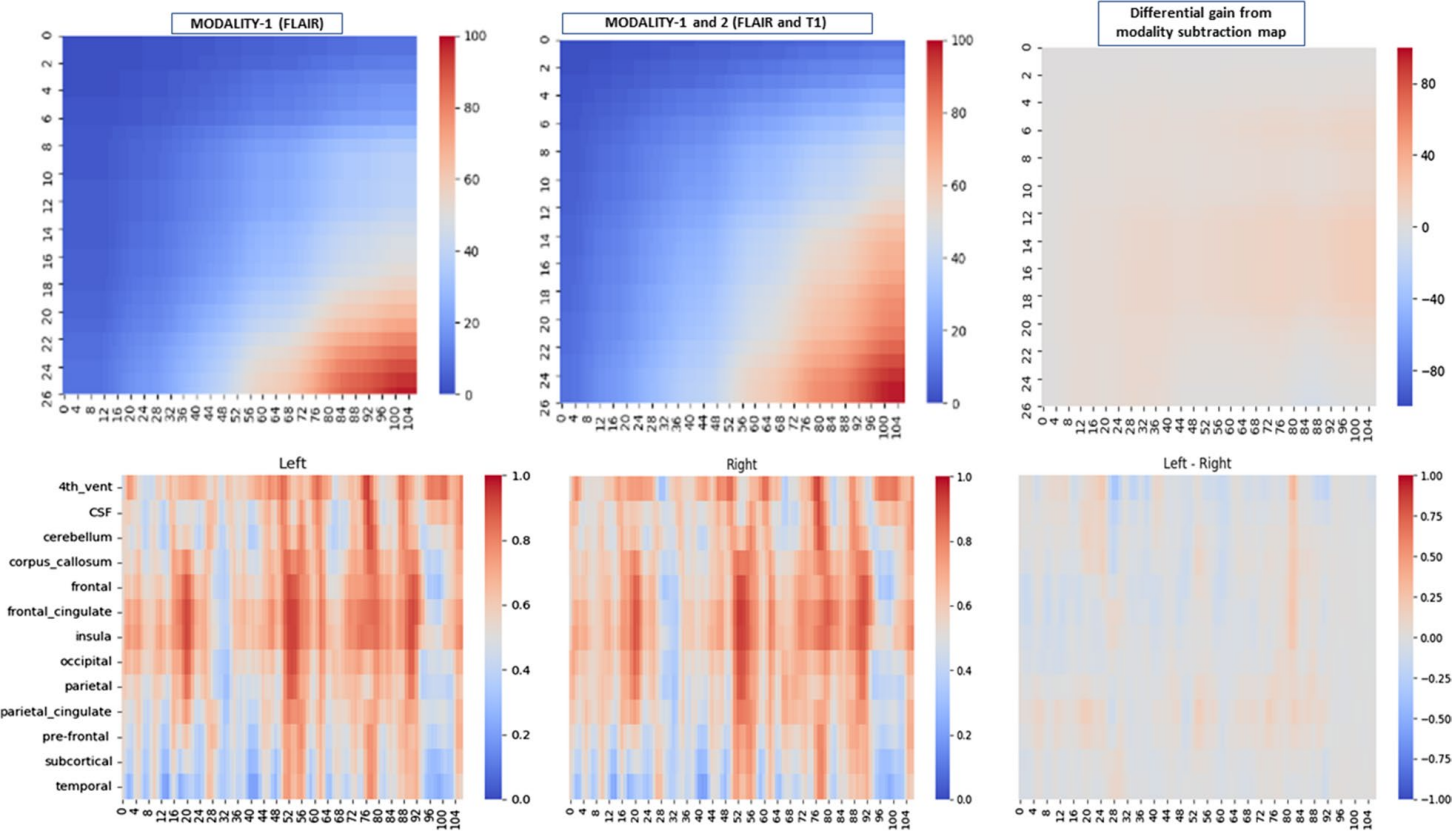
Top: Combined MRI modalities in Gauss filtered bicluster maps. Left: single modality. Centre: two combined modalities. Right: effects of modality subtraction (from two to one). Bottom: Visualization of differential effects induced by GBM in left and right hemispheres (N = 13 regions used to map) and by subtracting unsorted biclustering maps (rightmost plot). The MRI modality used is FLAIR
